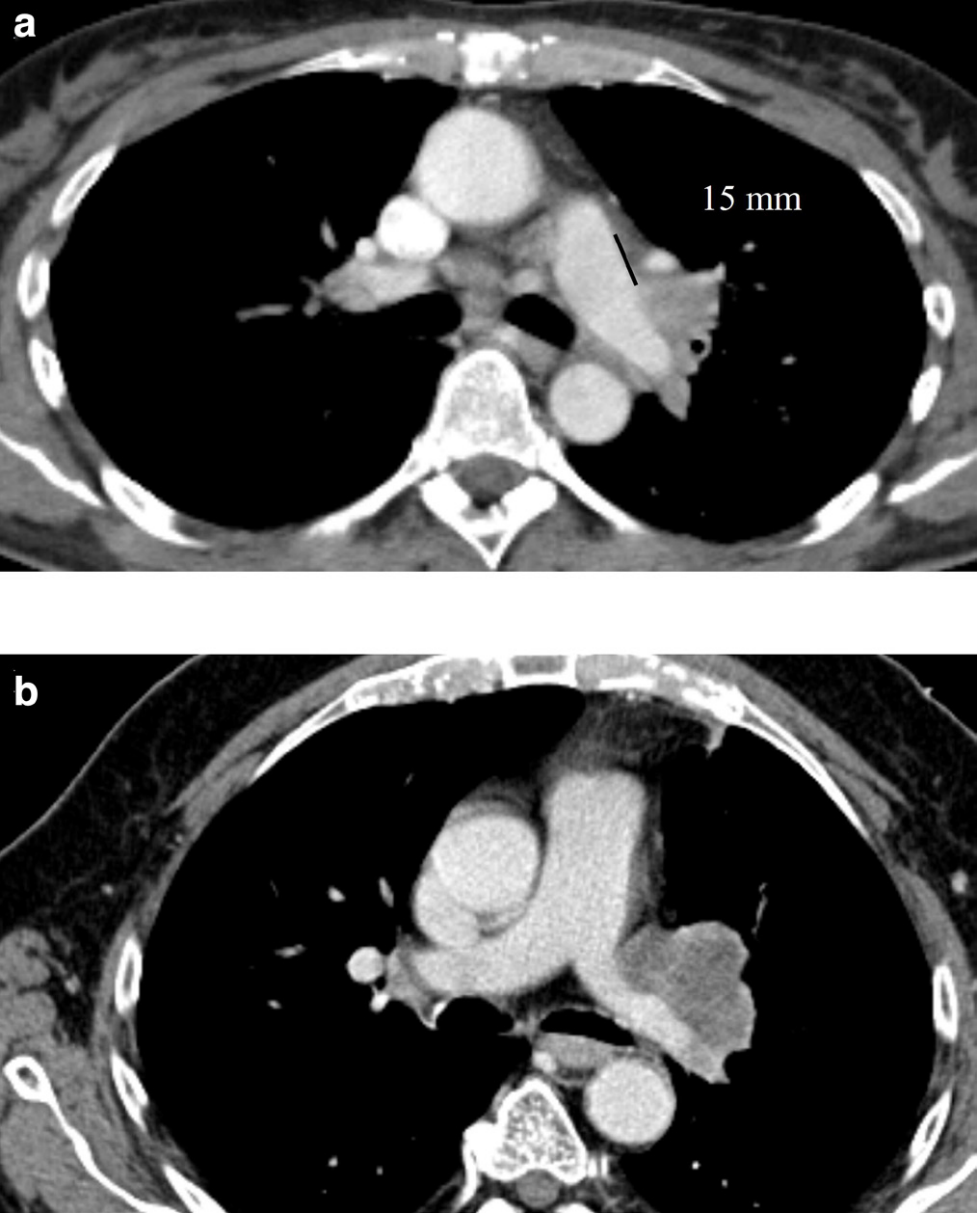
(a) Axial contrast-enhanced CT of a 55-year-old female patient with an NSCLC at the left hilum. As the distance between the main pulmonary trunk and the tumour is more than 10 mm, it is classified as T3 disease. (b) In contrast, in this 73-year-old male patient with NSCLC, the tumour is in direct contact with the main pulmonary artery and left main pulmonary artery, and therefore, staged as T4. NSCLC, non-small cell lung cancer.
